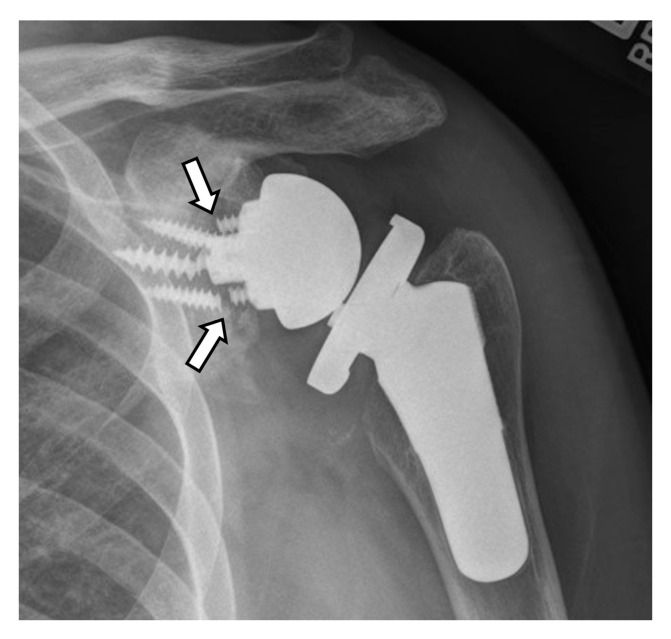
Frontal radiograph in a patient with an RTSA shows fracture/break (arrows) of three of the baseplate screws.
